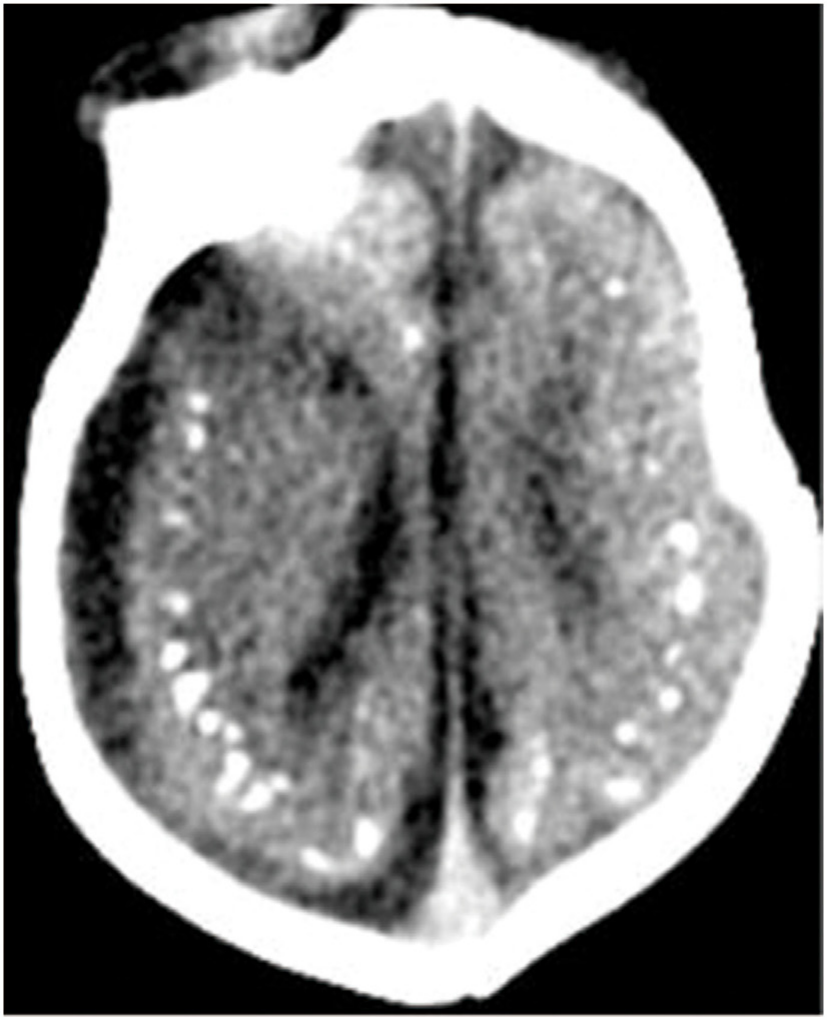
Axial CT image shows calcifications at the junction of the corticex and subcortical white matter, and parenchymal atrophy. All figures are courtesy of Lara Brandão M.D., Rio De Janeiro, Brazil.
